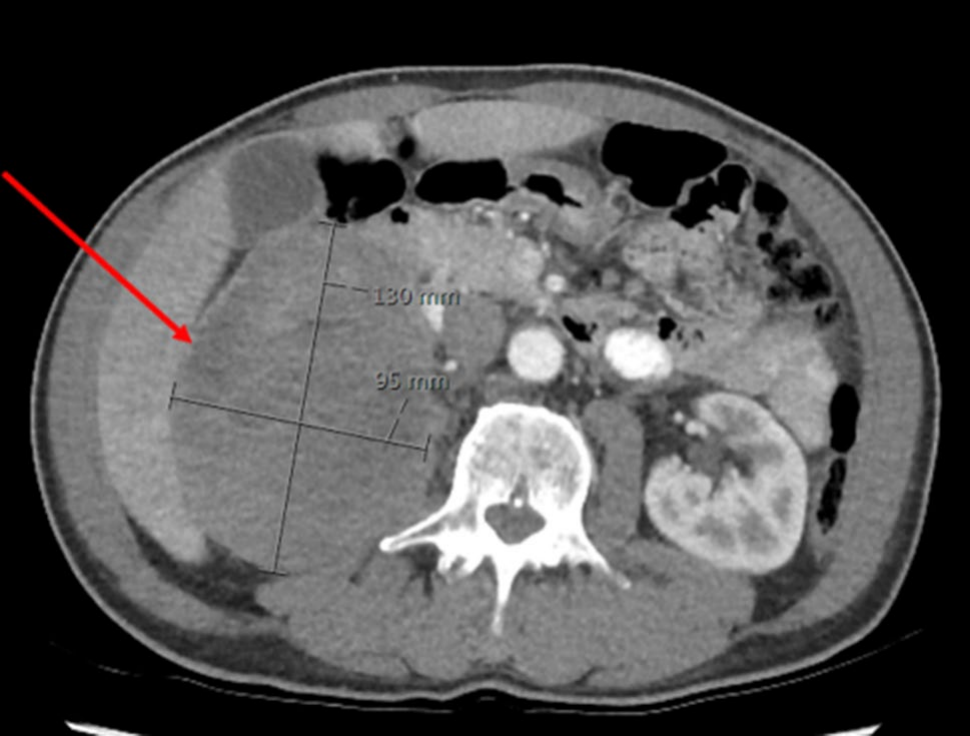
Abdominal CT scan demonstrating a mass of 13 cm originating from the right adrenal gland in patient 3
